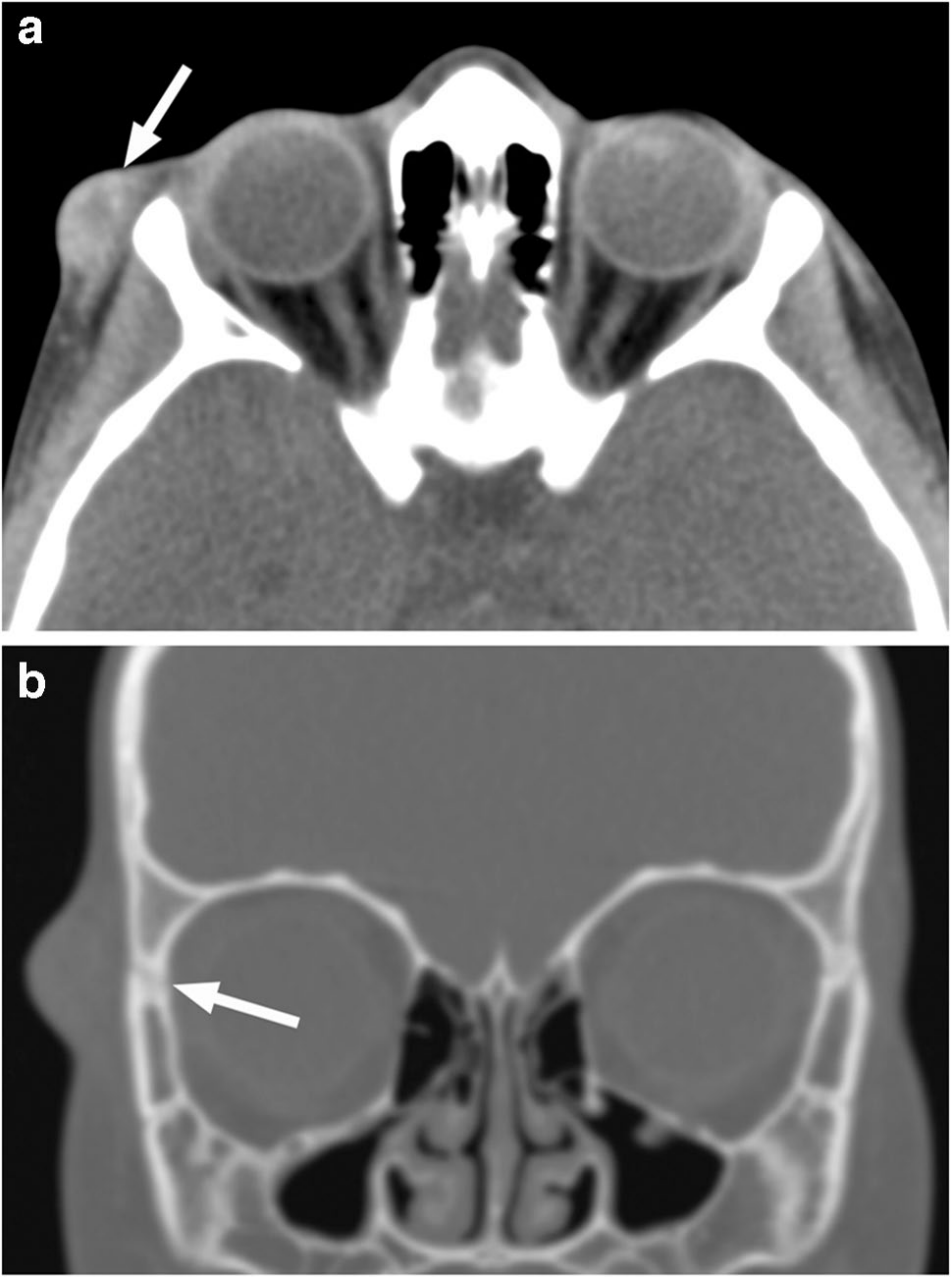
Nonenhanced axial CT image in soft tissue window (a) reveals a right periorbital slightly hyperdense oval subcutaneous mass with central hypodensity (arrow). Coronal CT image in bone window (b) reveals that the lateral periorbital swelling is adjacent to the right frontozygomatic suture (arrow) in this patient with dermoid
